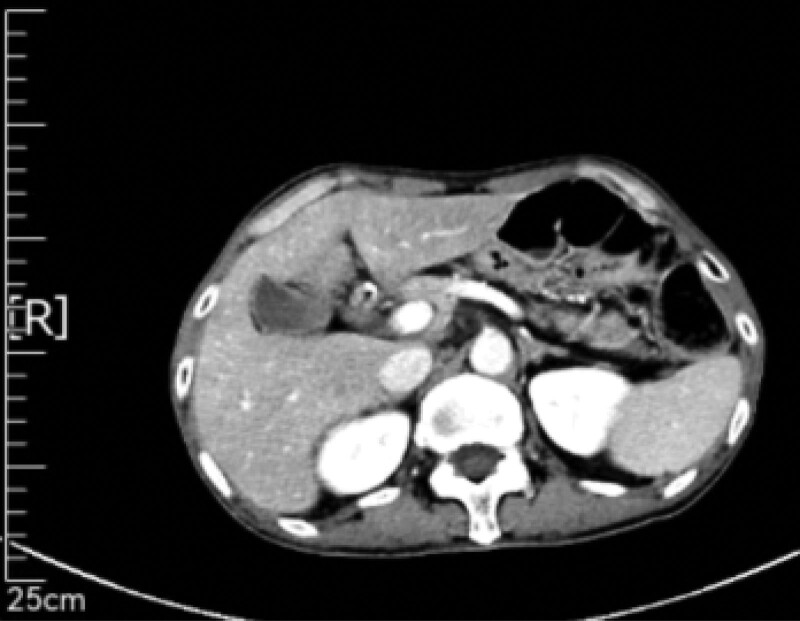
June 18, 2022, the CT examination results showed a decrease in liver S6 segment nodules compared to the old film of April 2, 2022. CT = computer tomography.
